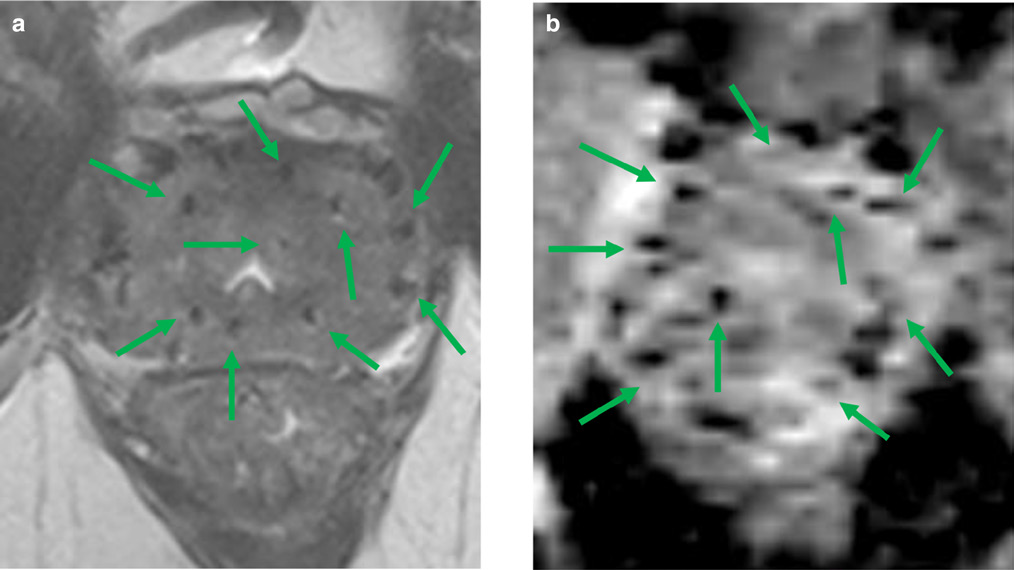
Imaging after brachytherapy MRI of a 68-year-old male with an initial PSA of 7.4 ng ml^−1^ after brachytherapy in 2012 as treatment for a Gleason score 3+4=7 lesion at the left peripheral zone. (a) Axial *T*
